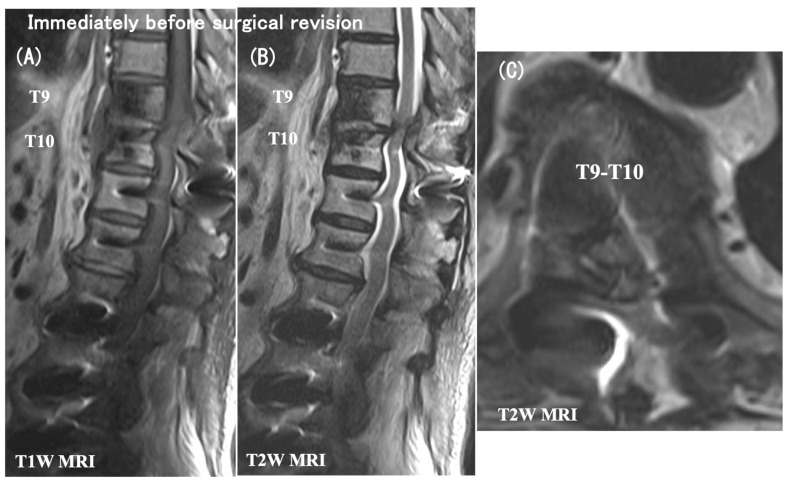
T1- (A) and T2-weighted (B) midsagittal MRI and a T2-weighted axial MRI at the T9–T10 intervertebral level (C) obtained immediately before surgical revision. These MRI scans showed distinct spinal cord compression at the T9–T10 intervertebral level (i.e., between UIV and UIV + 1).
